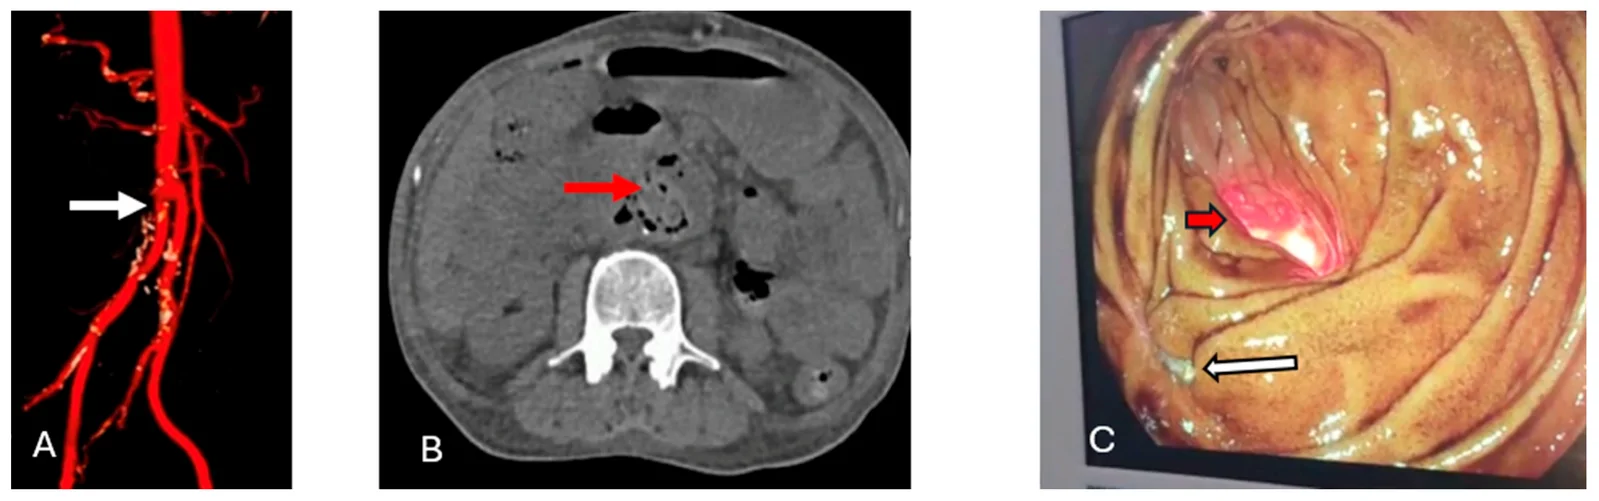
(Case 8): (A) Reconstructed CT scan with contrast (white arrow) indicating the graft following repair of rAAA with rifampin-bonded graft. (B) CT scan with contrast (red arrow) indicating air around the graft. (C) Intraoperative endoscopy: light of the endoscope in the third part of the duodenum (red arrow) and the tip of artery forceps (hemostats) introduced from the aneurysm sac to point to the fistula site (white arrow).
